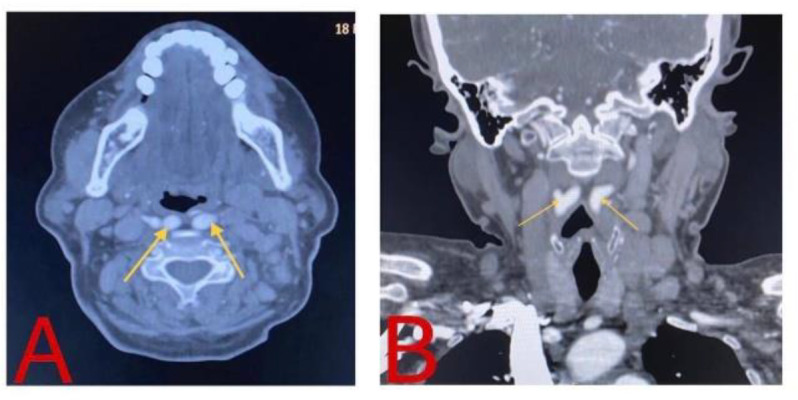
CT angiogram in axial (A) and coronal view (B), showing the medial course of the carotid artery giving the appearance of ‘kissing’ (yellow arrow).
